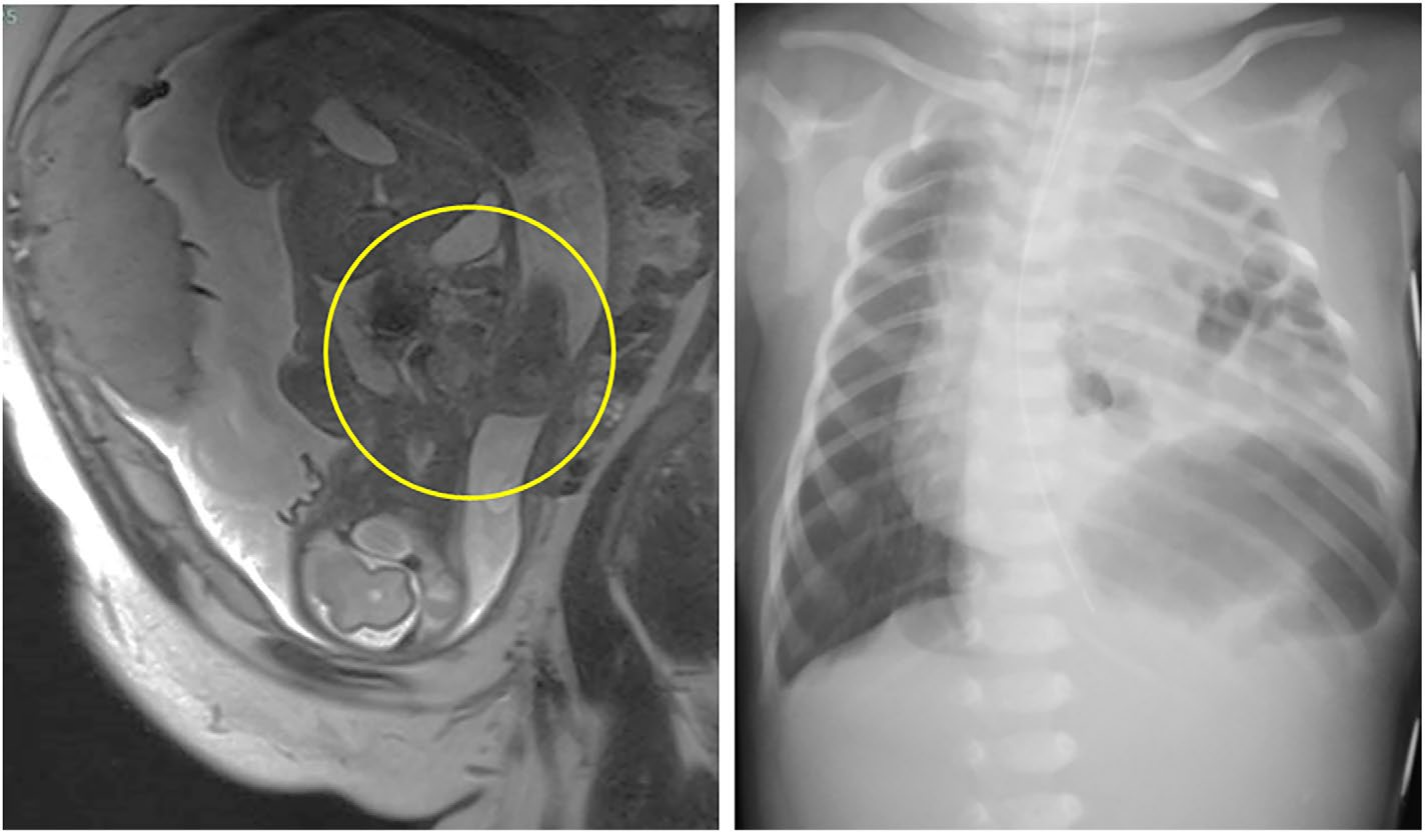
Image findings of fetal MRI and chest X‐ray at birth. Left: Fetal magnetic resonance imaging, right: Chest X‐ray at birth.
